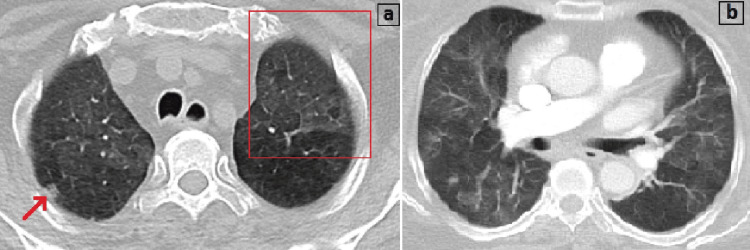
(a) The CT image shows a small nodule (red arrow) and a crazy-paving sign (red square), and (b) bilateral ground-glass opacities consistent with viral pneumonia.
